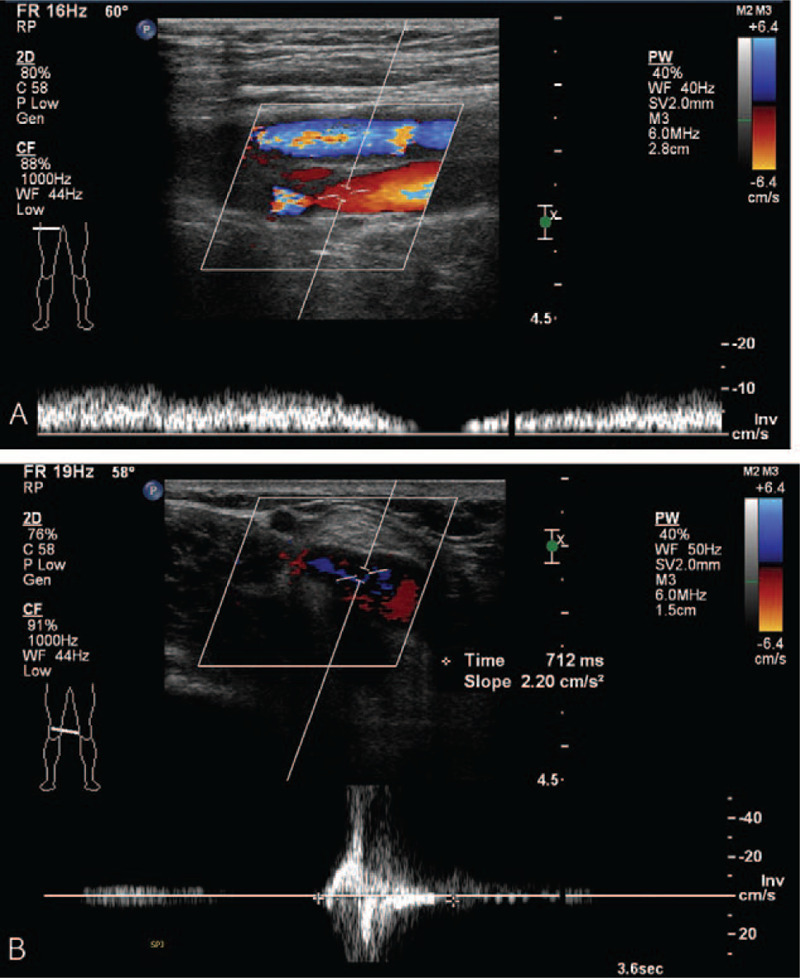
(A) & (B) Duplex sonographic findings of the venous system in the right leg.
